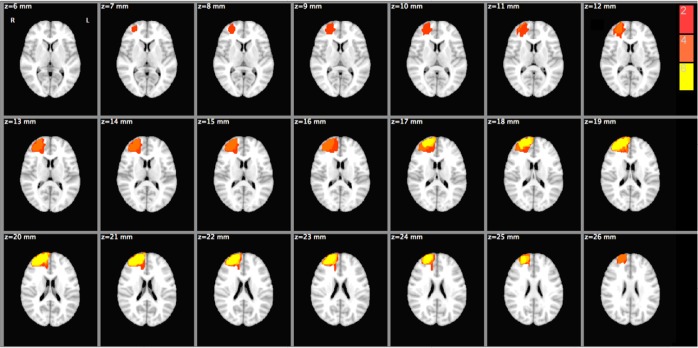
The neuroanatomical analysis of the brain lesions among six patients with the rFPC damage Representation of the damage to rFPC using MRI; The yellow color indicates the overlap between six patients, orange color represents overlap of the damage among four patients, and red color indicates overlap between damage areas among two patients.
